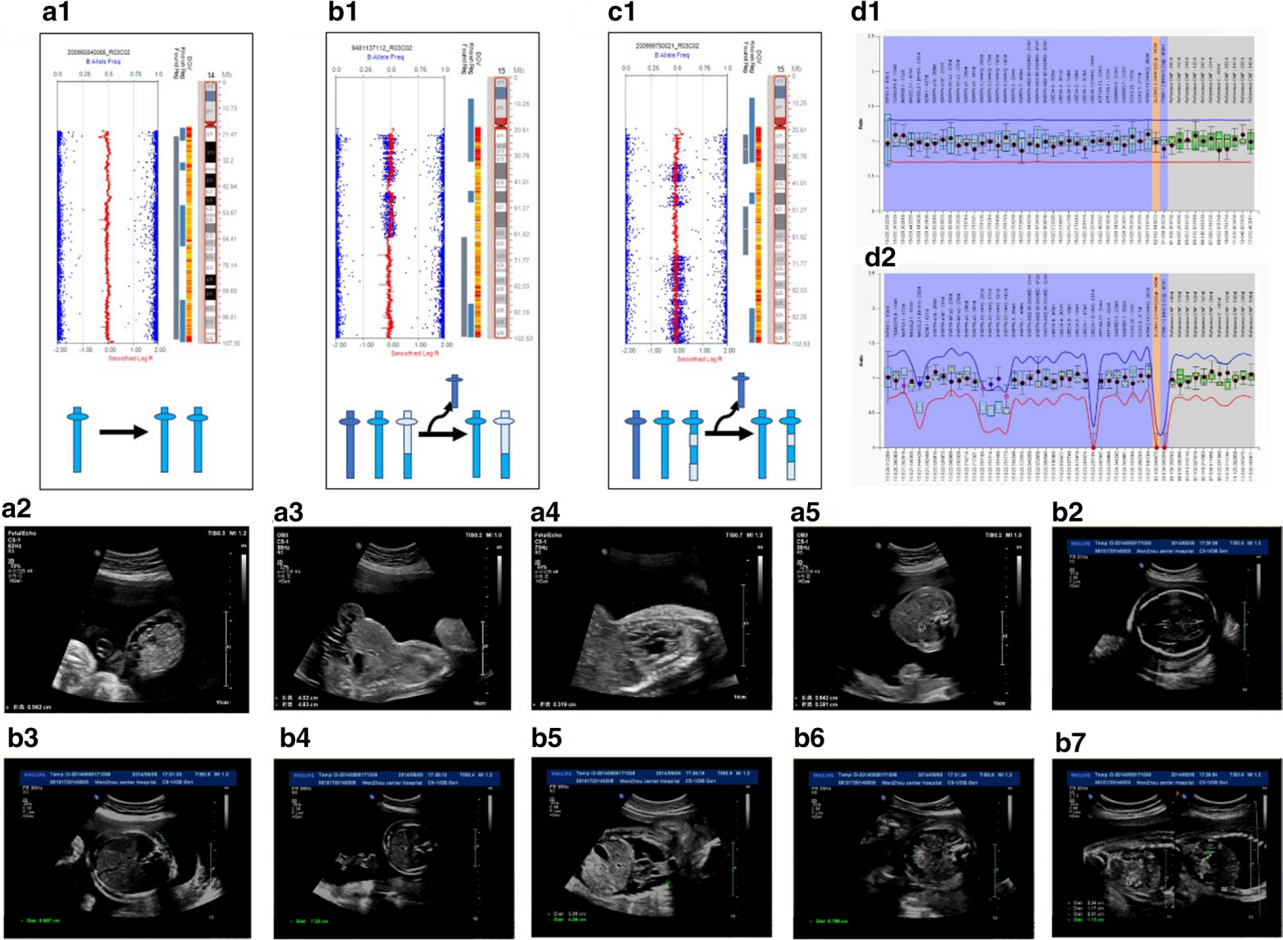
SNP array results and multiple ultrasonic malformations of three cases involved imprinted chromosome 14 and 15. (a1) SNP array revealed that case 3 arose by monosomy rescue with iso-UPD (14). (a2–a5) The multiple ultrasound malformations observed for case 3 included peritoneal effusion, omphalocele, ventricular septal defect, and small gastric vesicle. (b1) SNP array revealed that case 5 arose by trisomy rescue with mixed hetero/iso- UPD (15) from meiosis I non-disjunction error. (b2–b7) The multiple ultrasound malformations observed for case 5 included scalp edema, chest wall edema, abdominal wall edema, bilateral pleural effusion, ascites, and bilateral kidney edema. (c1) SNP array revealed that case 6 arose by trisomy rescue with mixed hetero/iso-UPD (15) from meiosis II nondisjunction error. (d1) The copy number ratio of the 15q11 region was 1 for two cases of UPD (15). (d2) Methylation ratio of the imprinted allele of chromosome 15q11 after digestion was 1 for two cases of UPD(15)
